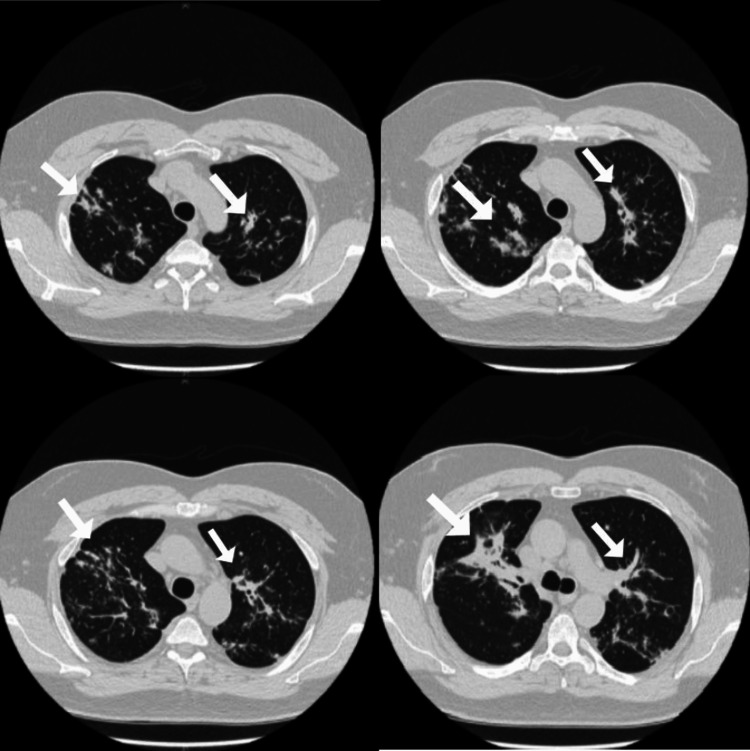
CT chest showing bilateral hilar lymph nodes with multiple irregular shaped nodules.
